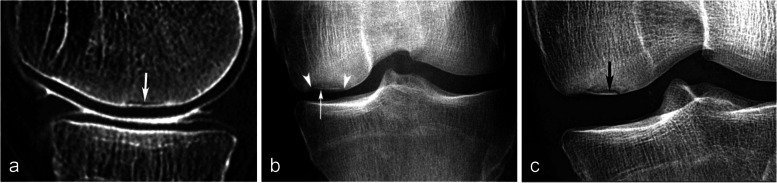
Radiological pattern of a “mobile” subchondral fracture, pathognomonic for necrosis. a On CT arthrography, a very thin fracture/separation in the subchondral trabecular bone appears as a radiolucent line (“eggshell dissection”) (arrow). b A few months later, a radiograph shows a collapse of the condyle lower pole (arrow) with breaks in the continuity of the subchondral bone plate (arrowheads). c An additional radiograph performed by fluoroscopy and with traction on the leg shows a slight opening of the subchondral bone dissection (arrow)
